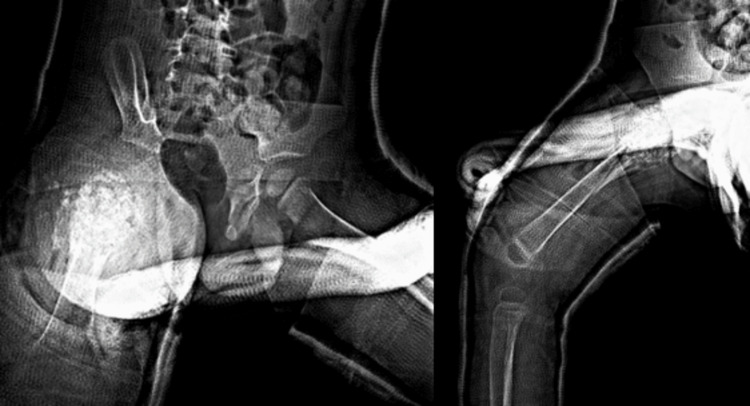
Anteroposterior and lateral radiographs intraoperatively
